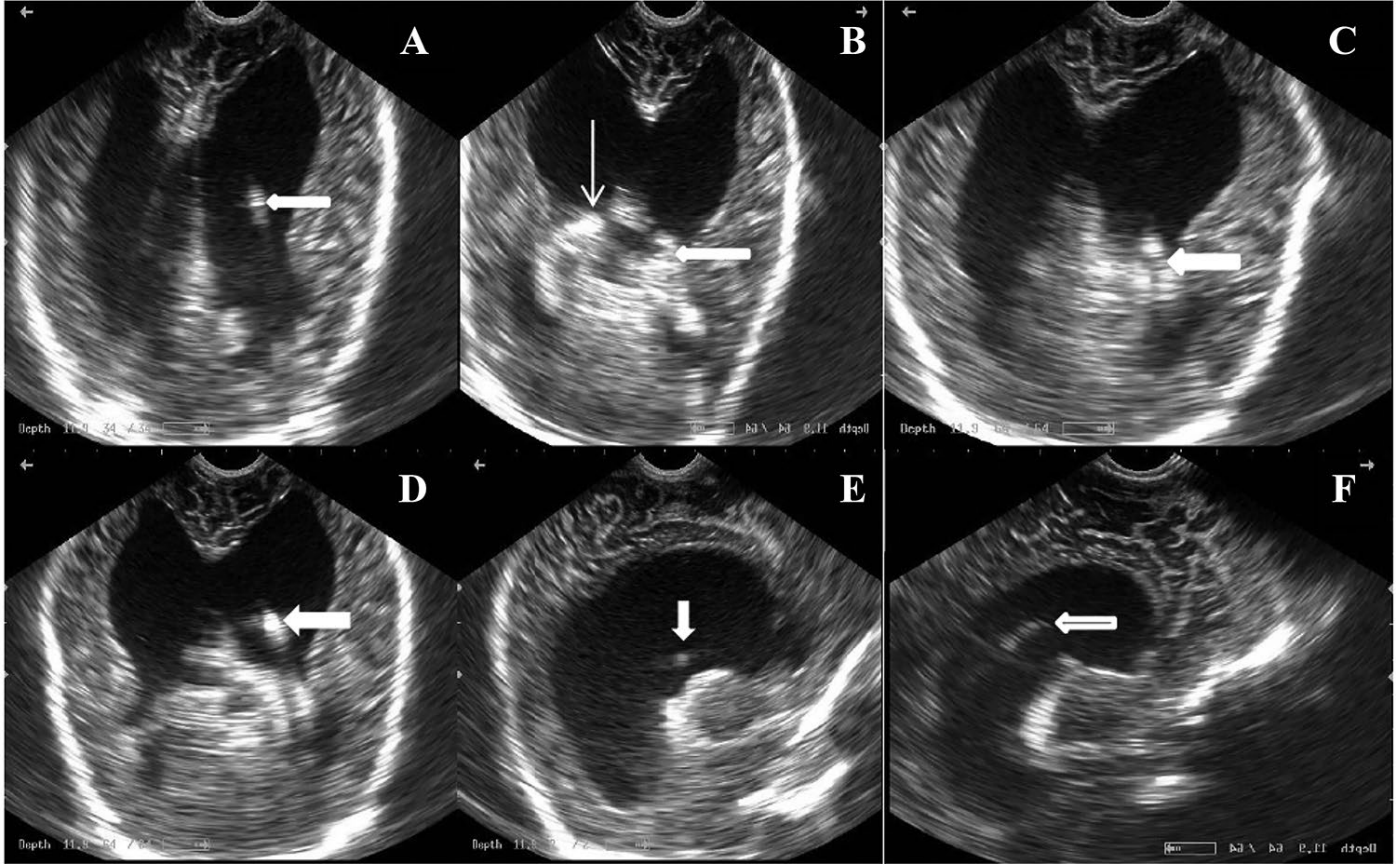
Steps of ultrasound guided cranial part insertion in the fontal horn; thick arrows point to the catheters and thin arrow points to the choroid plexus. A The catheter with the stylet in the body of the lateral ventricle, B identification of the choroid plexus, C finding the pathway to the frontal horn, D advance the catheter with the stylet to the desired level, E and F sagittal ultrasound views
